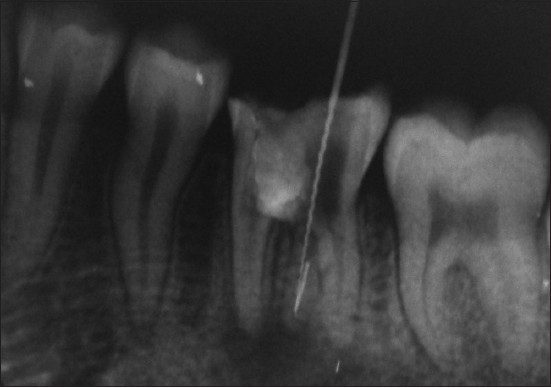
Radiograph showing inability to bypass the separated instrument
